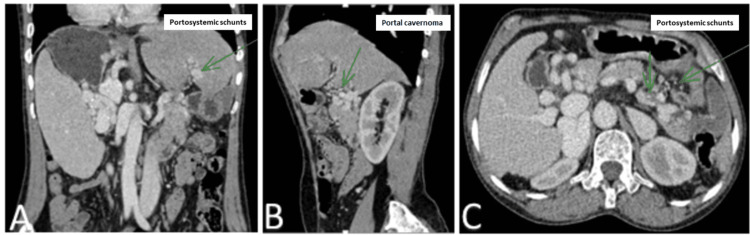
CT angiography images in axial (A), parasagittal (B), and coronal (C) sections showing a portal cavernoma with portosystemic shunts CT: computed tomography
